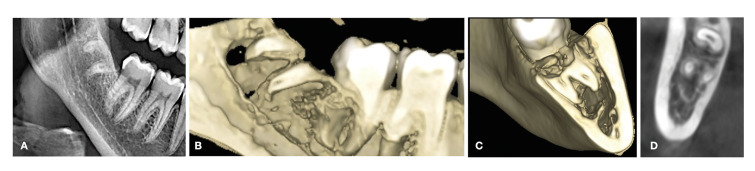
A) Panoramic radiographic study after 24 months of follow-up. The migration of the roots can be noted. B) CBCT reconstruction after 24 months of follow-up, showing the separation between the inferior alveolar nerve and the mesial root. C) CBCT reconstruction after 24 months of follow-up, showing the separation between the inferior alveolar nerve and the mesial root. D) Parasagittal view showing the separation between the apex and the inferior alveolar nerve.
